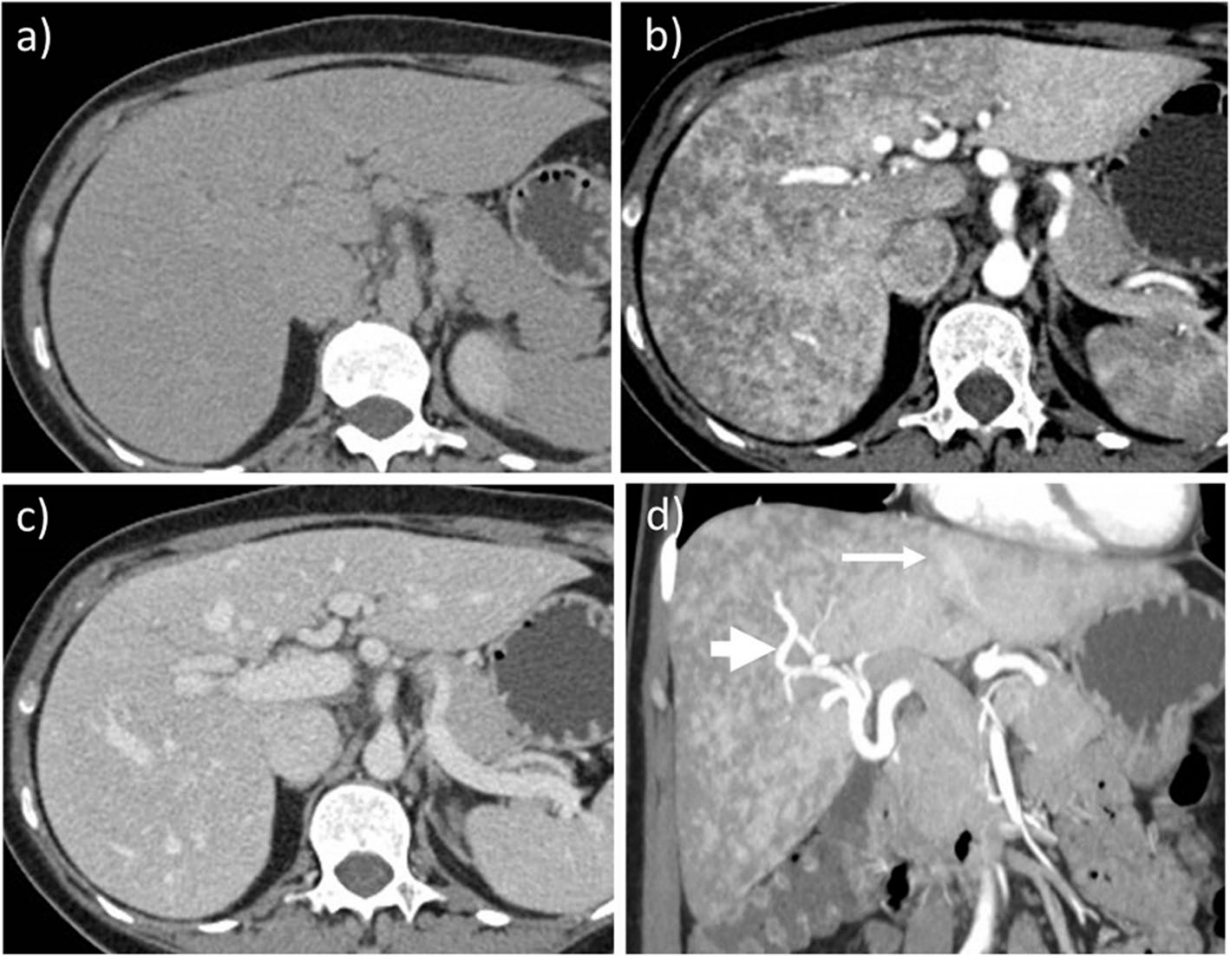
Hereditary hemorrhagic telangiectasia discovered in a 33-year-old pregnant woman. The patient had an episode of hemoptysis that led to a contrast-enhanced CT. Liver is slightly heterogeneous on unenhanced image (a). Enhanced CT shows the presence of a heterogeneously enhancing liver on hepatic arterial phase (b) with portal phase homogenization (c). Coronal plane MIP reconstruction on arterial phase (d) shows the presence of enlarged intra- and extrahepatic artery (> 6 mm—thick arrow) and early enhancement of left hepatic vein (thin arrow)
